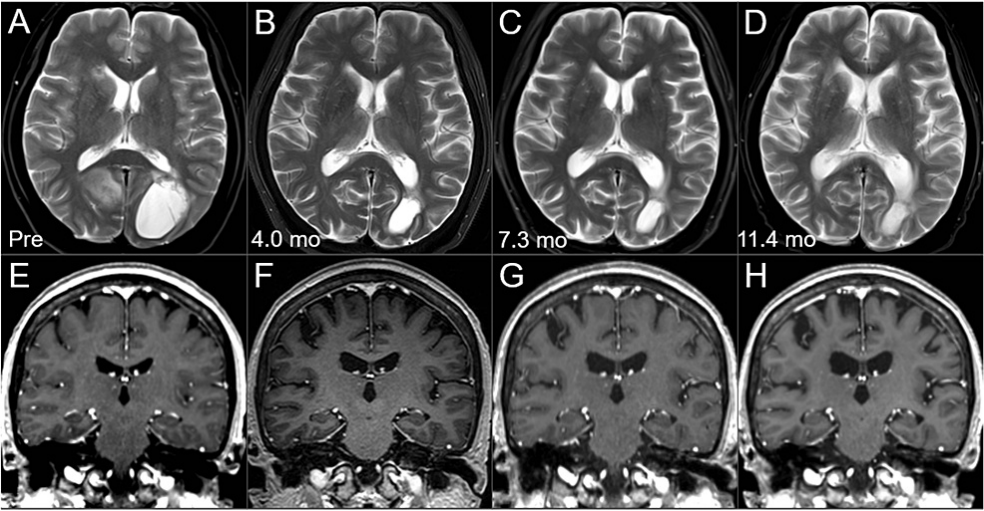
Morphological changes of the brain on magnetic resonance images before and after the external beam radiotherapy for brain metastases. The images show axial T2-WIs (A-D) and coronal CE T1-WIs (E-H); before the WBRT (pre, at age 57) (A, E); at 4 months after the WBRT (B, F); at 7.3 months (C, G); and at 11.4 months (D, H). (A-H) These images are shown at the same magnification and coordinates under co-registration and fusions based on the images before the WBRT. In particular, when comparing the images before and 11.4 months after the irradiation, ventricular dilatations, slightly high-intensity change in the periventricular deep white matters, widening of the cortical sulci, and the Sylvian fissures, suggesting the progression of parenchymal atrophy and degeneration, are observed, which are difficult to explain by tumor shrinkage alone. WI: weighted images; CE: contrast-enhanced; WBRT: whole-brain radiotherapy
